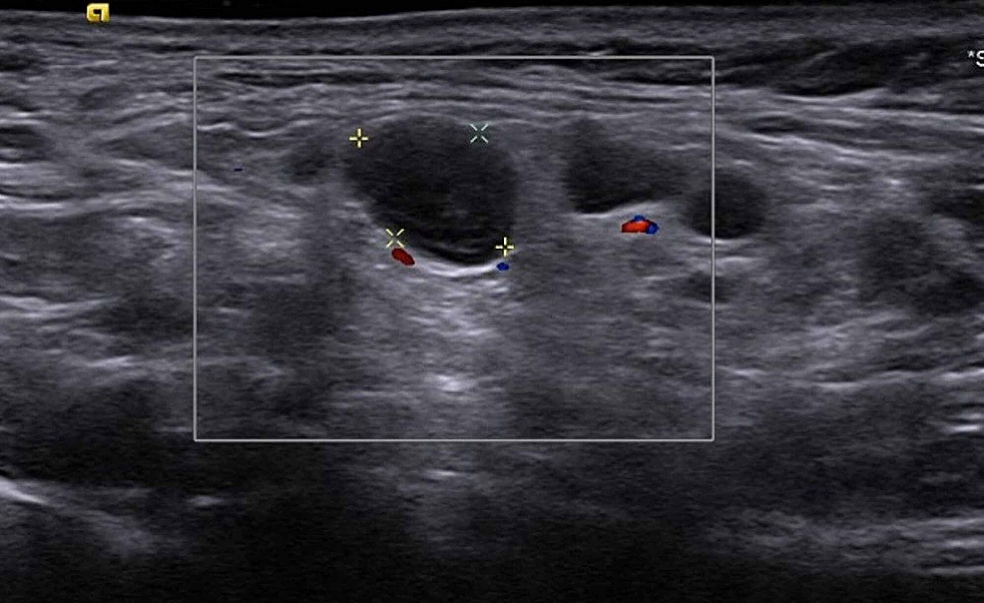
Ultrasound image of the supraclavicular area demonstrating hypoechoic rounded lymph nodes without identifiable hilum and without visible blood flow.
